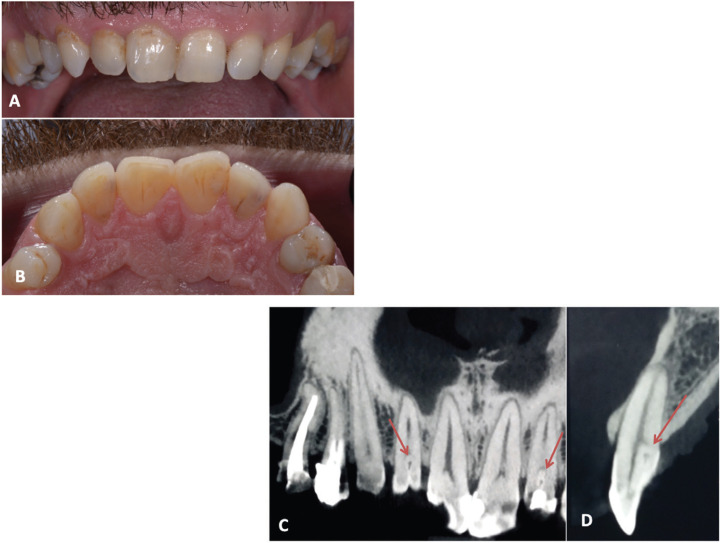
Photographic buccal (A) and palatal (B) view of maxillary teeth. CBCT images (C. frontal view, D. sagittal view) of maxillary teeth. The internal structure is characteristic of a dens invaginatus type II (Ohler’s classification).
